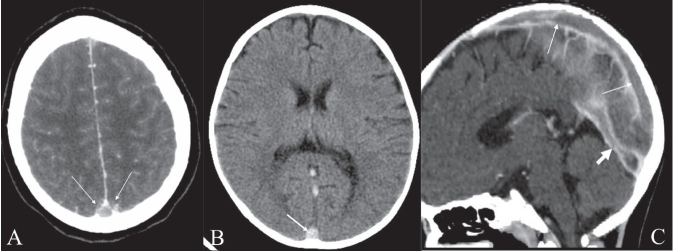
Empty delta sign. Postcontrast axial CT scan of the brain (A) shows the nonenhancing lumen of the superior sagittal sinus surrounded by enhancing dura (arrows). Nonenhanced axial CT scan of the brain (B) shows a hyperdense superior sagittal sinus (arrow) suggestive of thrombus within. This is called the ‘triangle sign.’ Sagittal reconstruction of a contrast-enhanced CT scan (C) shows filling defects in the superior sagittal sinus (long arrows) and in the straight sinus (thick arrow), suggestive of thrombus
